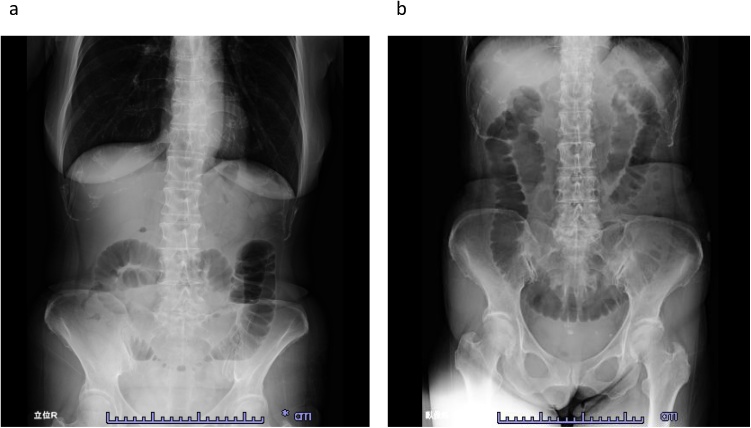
X-ray of the abdomen. a: Upright position. b: Seated position.
